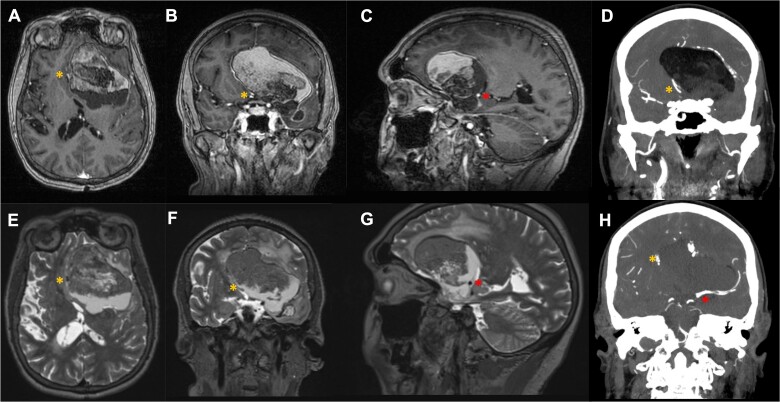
Pre-operative MR T1 post gadolinium [axial (A), coronal (B), sagittal (C)], coronal CT angiogram (D, H) and T2 [axial (E), coronal (F), sagittal (G)] demonstrating the vascular relationship of the left Sylvian fissure-centered dermoid with the anterior cerebral artery (orange *) and middle cerebral artery (red *) seen displaced by the lesion. There is surrounding edema (E,F,G) and heterogeneity in contrast enhancement (A,B,C), as well as a fluid level (A,B,C,E,F,G).
